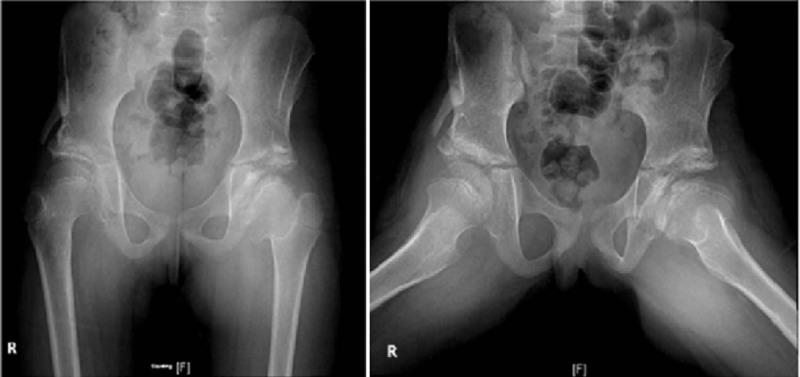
Patient with bilateral developmental dysplasia of the hip 7 years after novel treatment of the right hip: (A) Anteroposterior radiograph of the pelvis and (B) lateral frog view radiograph showing proper reduction and acetabular coverage.
